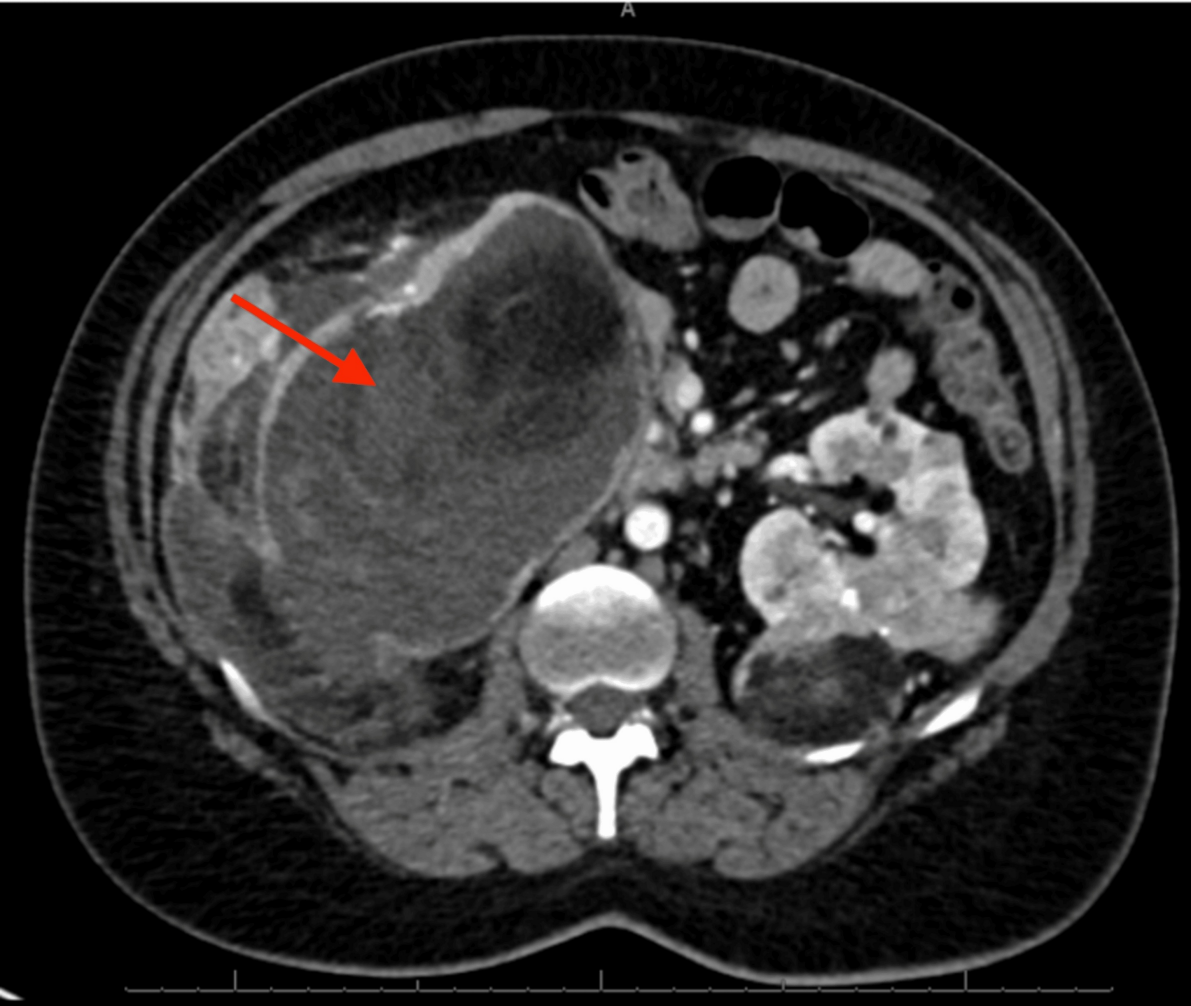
CT abdomen and pelvis: a right renal angiomyolipoma with no recognizable renal parenchyma measuring 16.1 x 12.0 x 19.6 cm with a right perinephric hematoma in the axial plane
